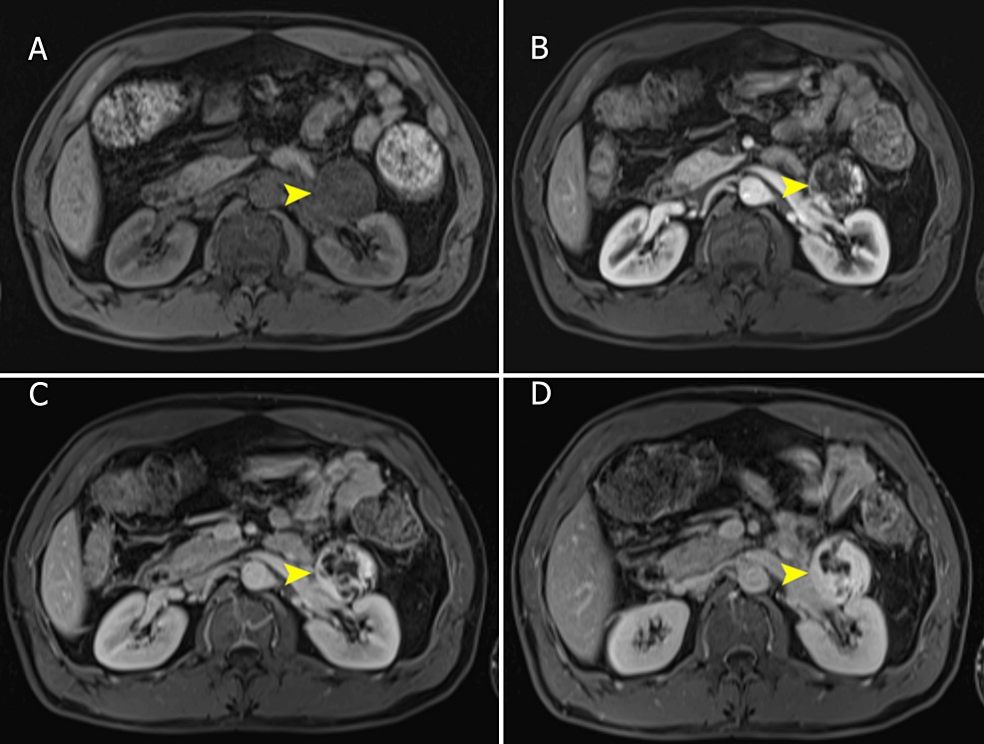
Abdominal MRI part 2, dynamic sequences Simple-phase fat-suppressed T1-weighted MRI sequence (A) and dynamic sequences after intravenous contrast medium administration (B, C, and D) show a low-signal-intensity mass in the simple phase and an avid, nodular, peripheral, and discontinuous enhancement in the corticomedullary phase, which is progressive and centripetal over time. The size of the lesion remained stable compared to the previous CT.
